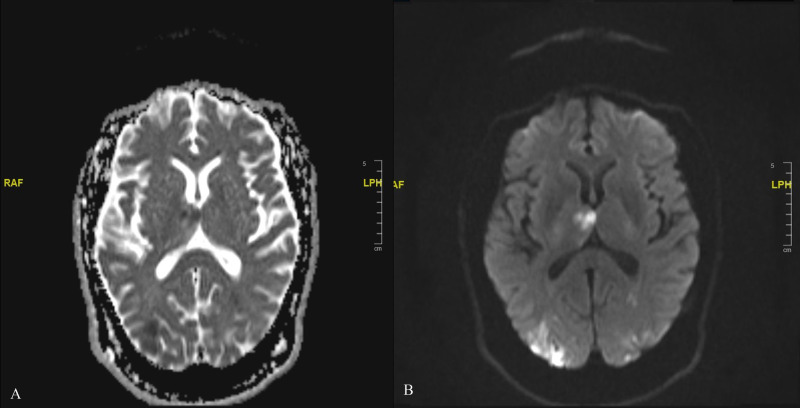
MRI of the brain of showing multiple embolic stroke (black arrow). (A) Hypointensity signals in an apparent diffusion coefficient (ADC) image. (B) Hyperintensity signals in a diffusion-weighted image (DWI).
